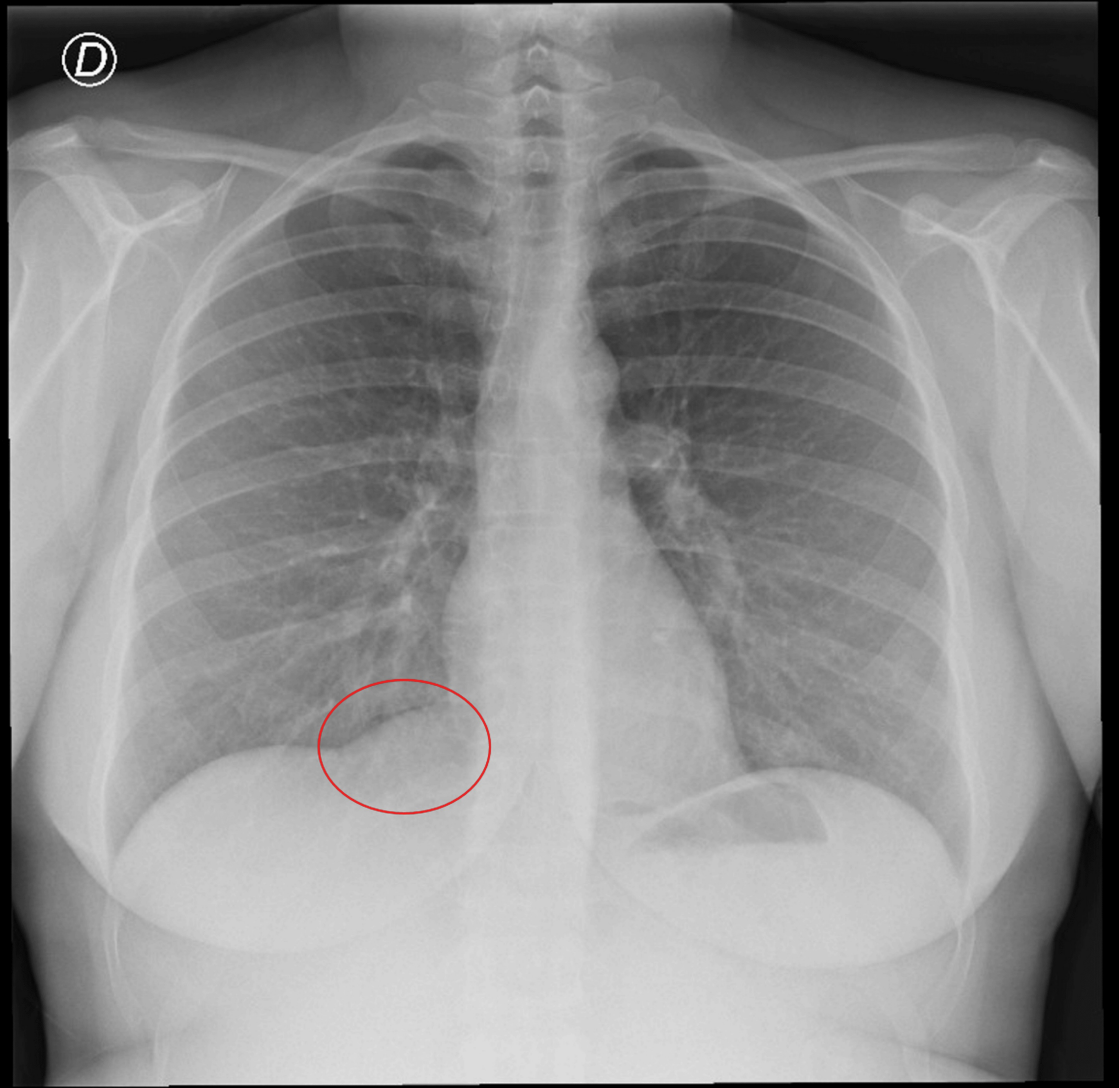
Chest X-ray of the patient shows a slight elevation of the right cardiodiophrenic angle (red circle)
